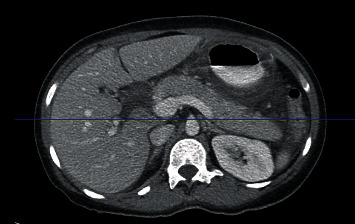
CT abdomen image obtained in Emergency Department indicating findings consistent with pancreatitis.
